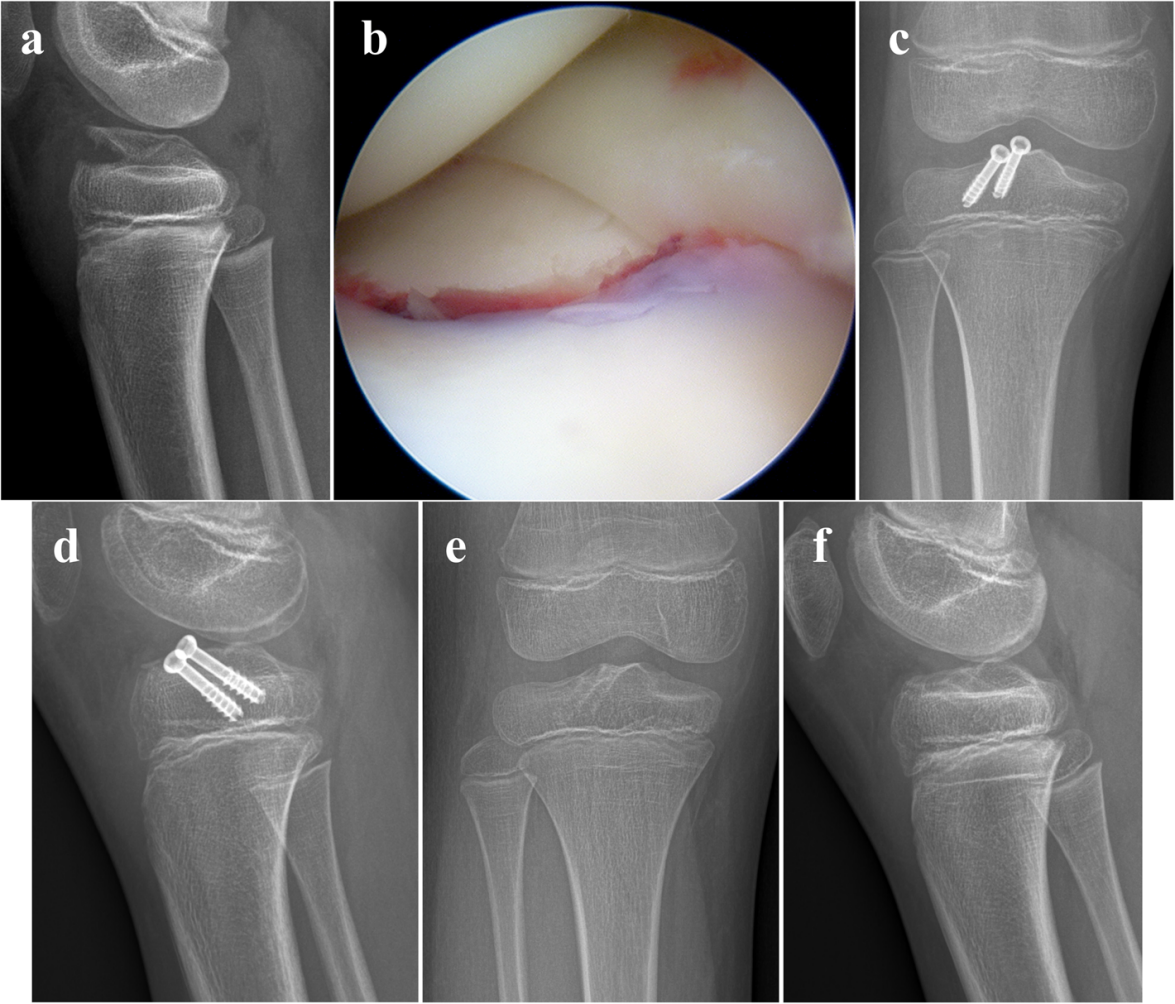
a A radiograph of a 10–11 year-old girl with tibial eminence fracture. b Anterior horn of medial meniscus entrapped under the avulsed fragment. c, d Radiographs taken at postoperative one month. The avulsed fragment was fixed with two 5.0 mm cannulated screws. e, f Radiographs taken at postoperative one year. Screws were removed. Her Lysholm knee screw was 100. She did not have instability of the knee
